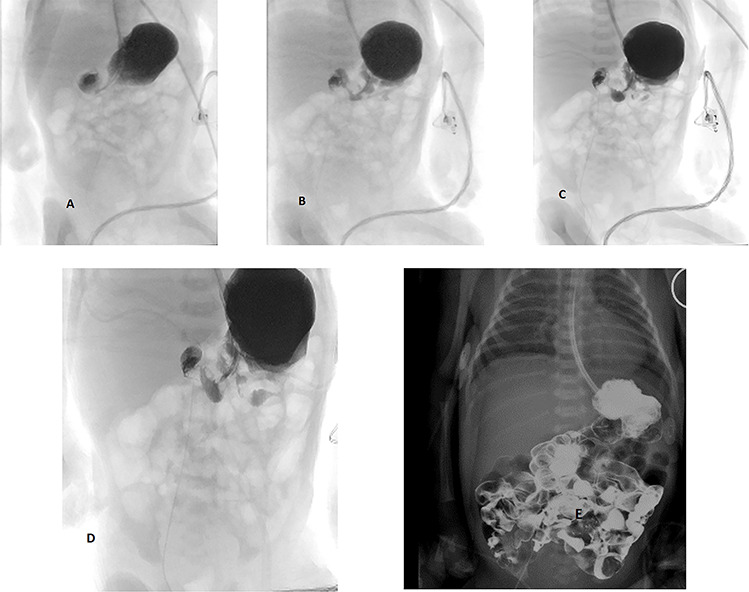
(a–c) Normal upper GI series; (d, e) normal upper GI series and final X-ray showing normal distribution of contrast throughout the small and large bowel.
